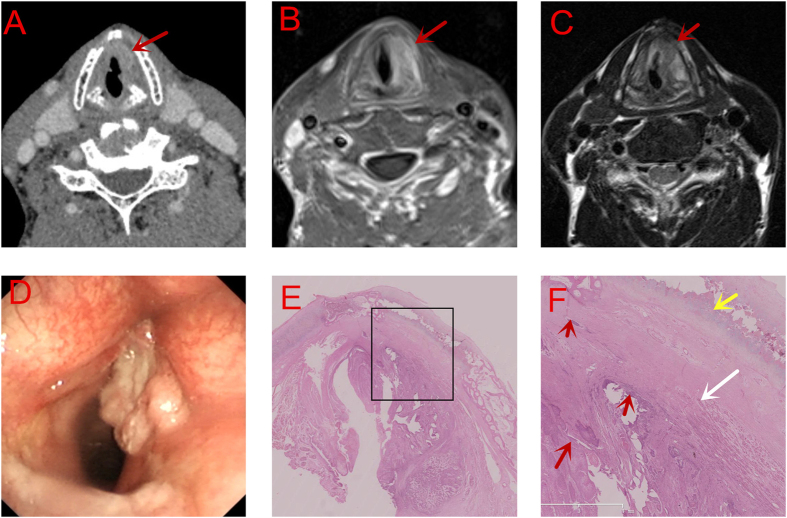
Thyroid Cartilage Uninvaded. (A) CT scan: Without involvement of thyroid cartilage (red arrow) (B).(T1WI)and (C).(T2WI) MRI scan: Involvement of thyroid cartilage (red arrow) (D). Electronic laryngoscopic examination. (E,F) (amplified image within the rectangle in E., 40×) Serial section: Without involvement of thyroid cartilage (Red arrow: tumor cells; Yellow arrow: the thyroid cartilage ;White arrow: the vocal muscle).
